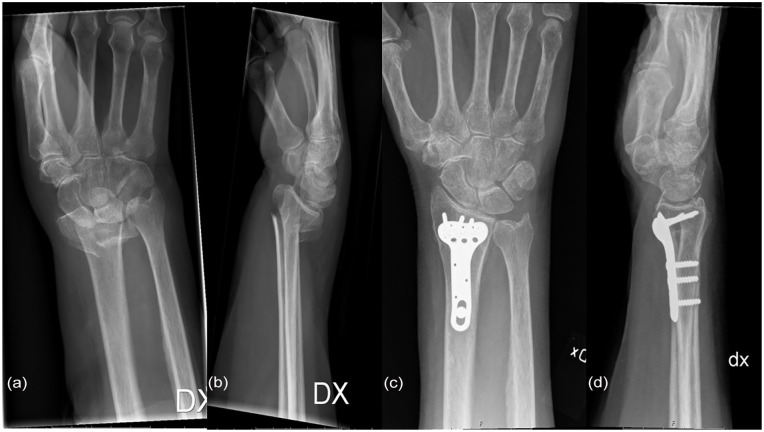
(a) Anteroposterior view of preoperative radiograph. (b) Lateral view of preoperative radiograph. (c) Anteroposterior view of 12-month postoperative radiograph. (d) Lateral view of 12-month postoperative radiograph.
